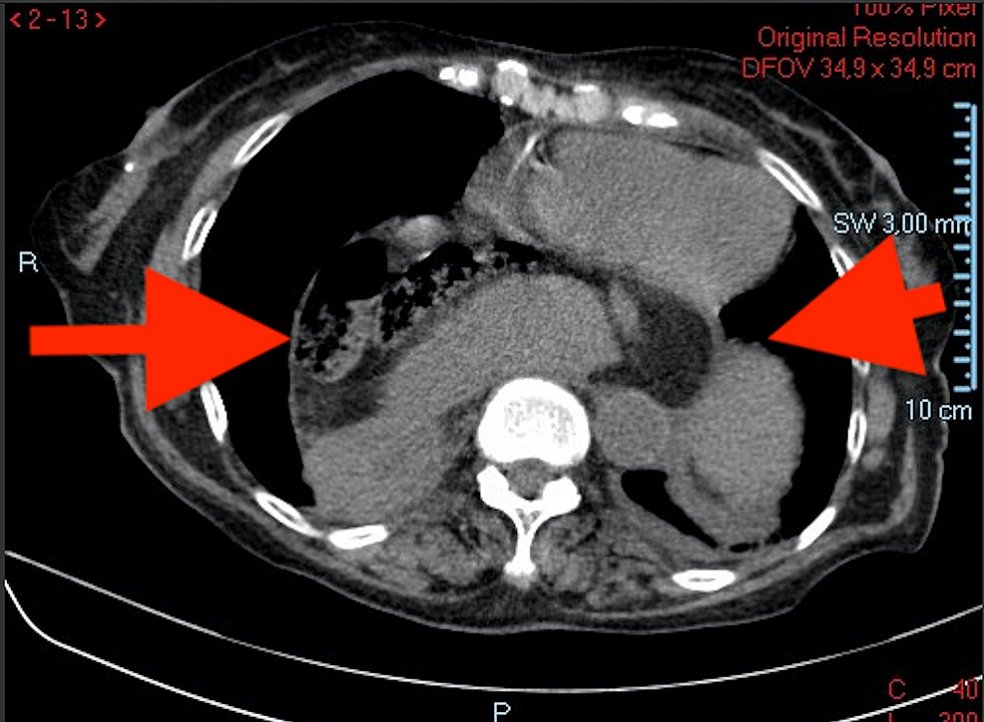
Transverse segment of a CT scan showing a distended stomach, with a suggestion of gastric volvulus in the intrathoracic segment. The arrows show the stomach within the thoracic cavity.
